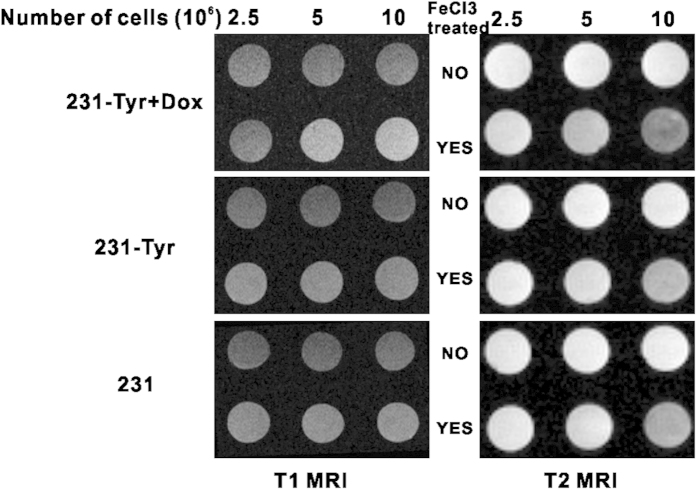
(A) T1 MRI (left) and T2 MRI (right) images of three concentrations of 231-TYR + Dox, 231-TYR and 231 cells pre-treated without (top row) or with (bottom row) FeCl_3_.
